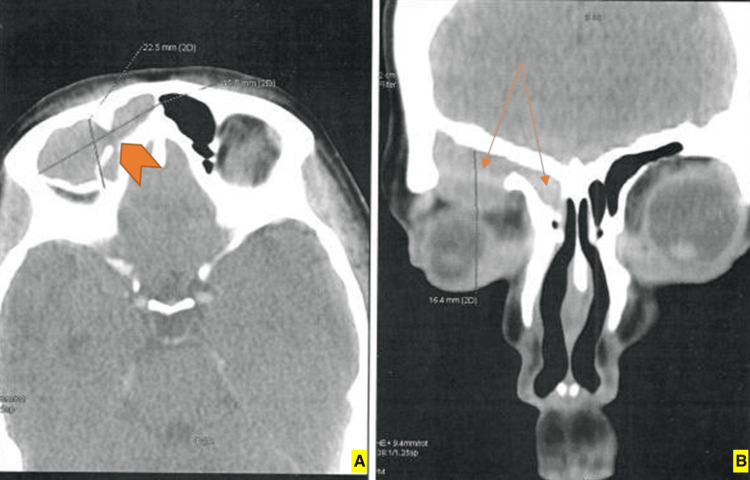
Computed tomography findings. (A) Coronal CT image demonstrating erosion of the anterior portion of the orbital roof (arrowhead). (B) Axial CT image showing an ethmoido-orbital fluid-filled lesion (arrow) with orbital extension. White arrow indicates the ethmoido-orbital fluid-filled lesion (mucocele). White arrowhead indicates erosion of the anterior orbital roof.
